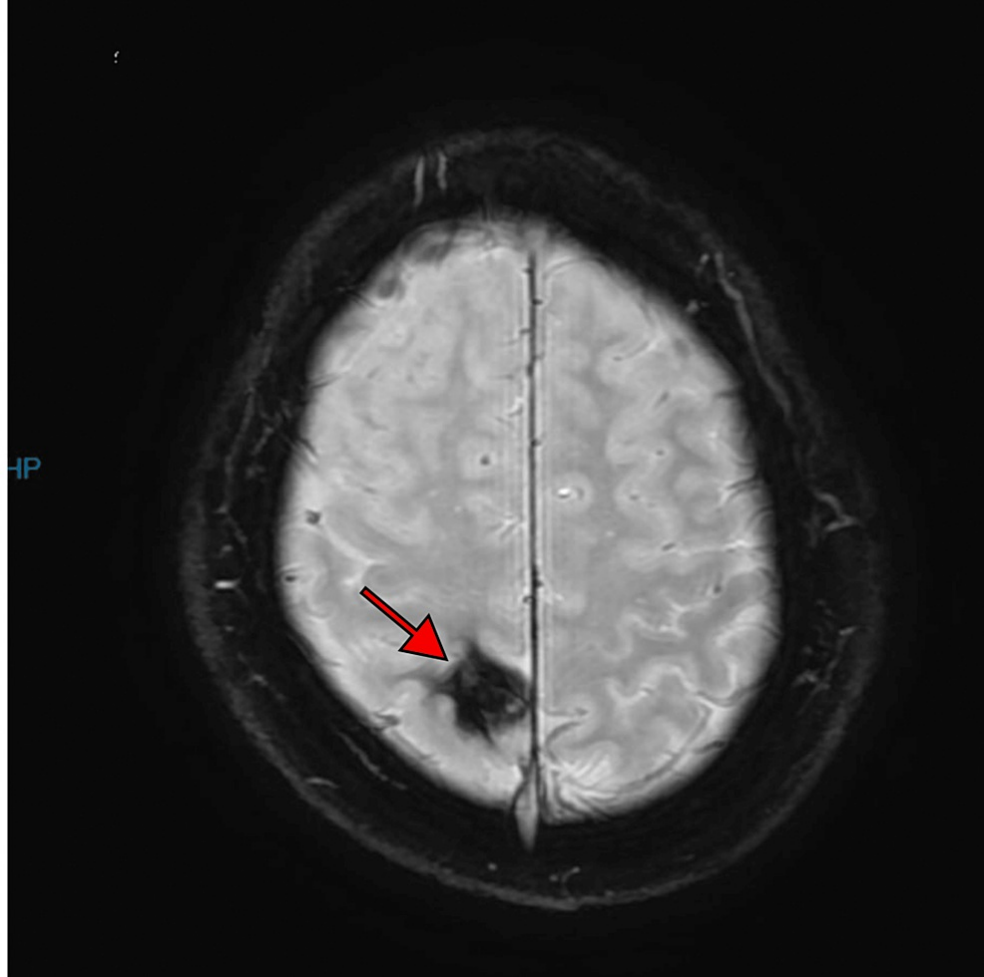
MRI axial view susceptibility-weighted image (SWI): the right parietal lesion shows a blooming artifact representing hemorrhage
